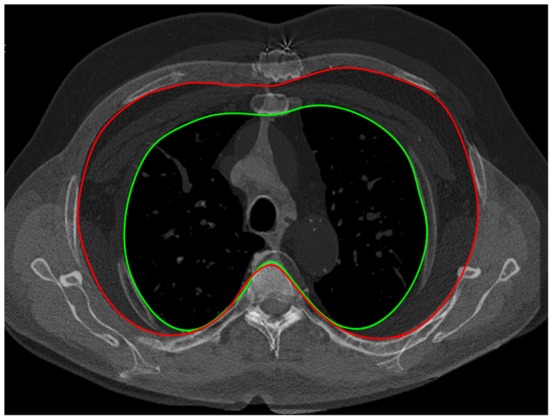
Example showing the independent effect of emphysema. Two overlapping upper (second level) CT slices of two different male subjects with similar demographic characteristics: the subject with the smaller area (green line) had an emphysema extent of 2.1%, whereas the one with the larger area (red line) had an emphysema extent of 35%.
